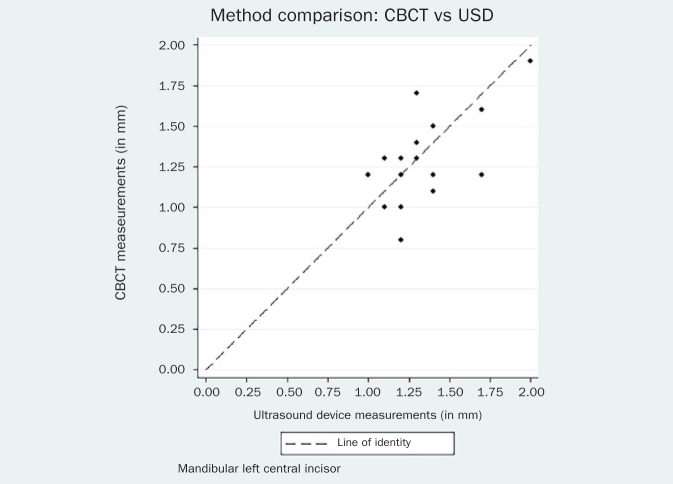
Method agreement plot CBCT vs USD 31.
